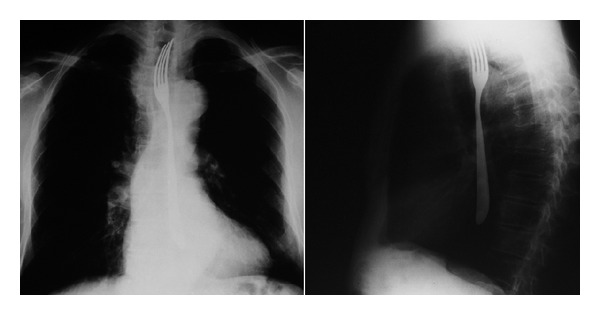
Antero-posterior and lateral X-rays of the thorax showing the foreign body (a table fork) occupying the entire thoracic esophagus.
